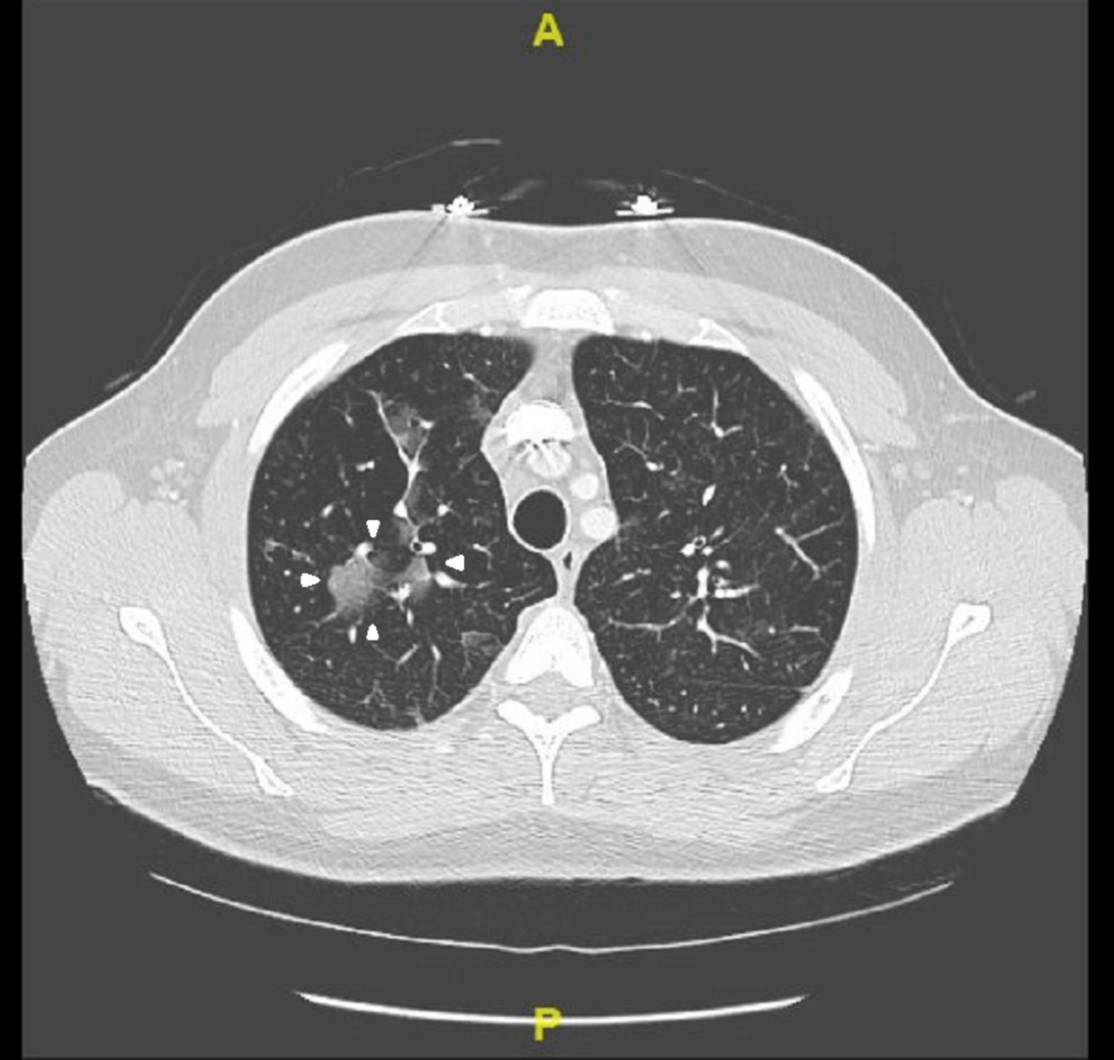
CT Angiogram of Chest with contrast
